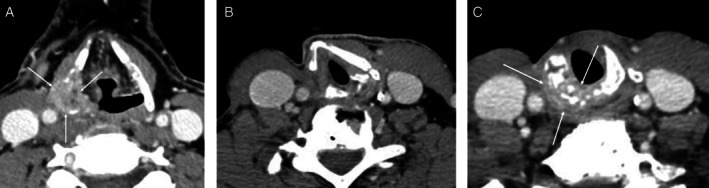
(A) Preoperative CT neck with contrast axial cut demonstrating a 2.6 cm tumor (arrow) involving the right thyroid cartilage and extending into the paraglottic space. (B) CT neck with contrast axial cut 9 months after surgery demonstrating right thyroid cartilage defect, without evidence of disease. (C) CT neck with contrast 2 years after surgery demonstrating disease recurrence in the right cricoid cartilage (arrow).
